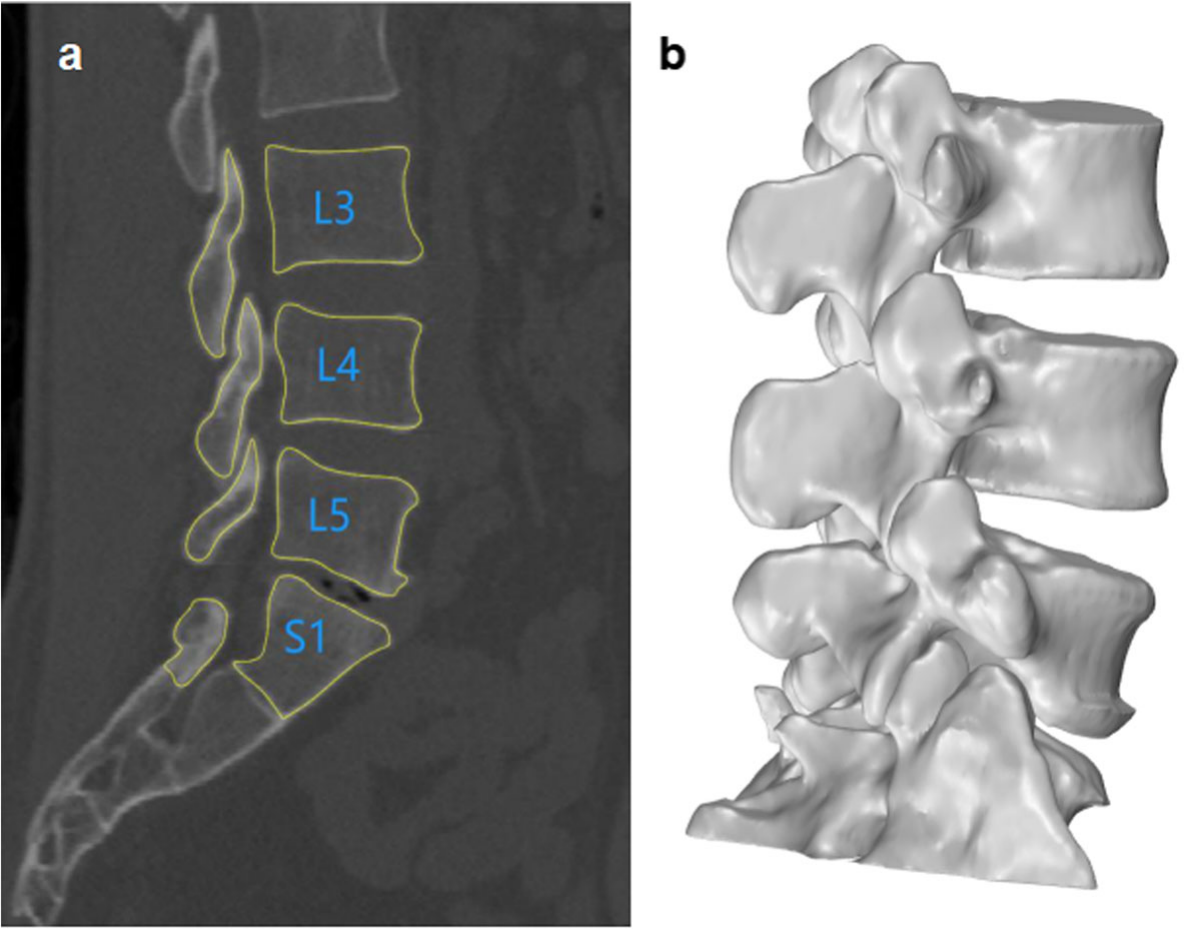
a A CT image of a human lumbar spine in the sagittal plane with segmentation lines present. b A 3-dimensional vertebral model from L3 to S1 constructed using CT images
